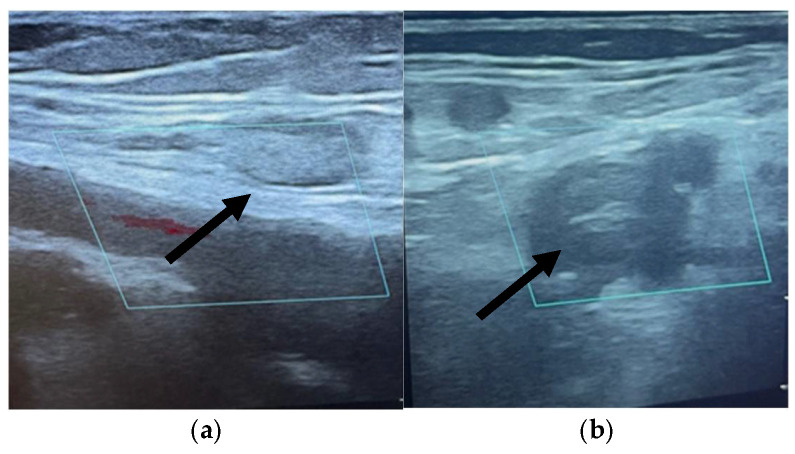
Ultrasound imaging reveals a complete (100%) thrombotic occlusion within the venous system, as demonstrated by the black arrows. (a) Ultrasound demonstrates a complete occlusive venous thrombus, highlighted by the arrow. (b) Arrow indicates the presence of an iliofemoral thrombus on ultrasound.
